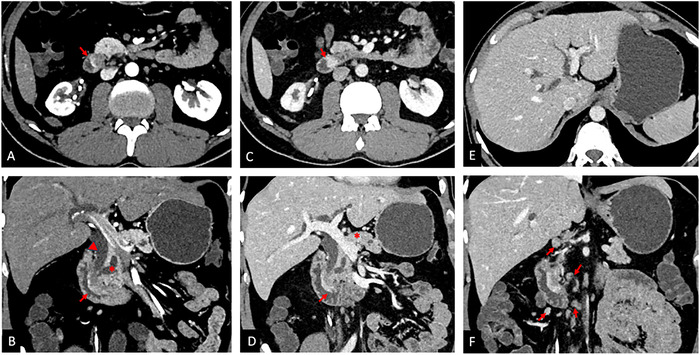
Contrast-enhanced computed tomography (A) axial reformatted image in pancreatic phase shows an ill-defined mass in the ampullary region with peripheral rim enhancement (arrow). (B) Coronal reformatted image in pancreatic phase shows a smooth bulge into the medial wall of the second part of the duodenum (arrow), as well as cutoff of the distal common bile duct (arrowhead) and pancreatic duct (asterisk) with upstream dilatation (the double duct sign). (C) Axial and (D) coronal reformatted images in portal venous phase show progressive enhancement of the mass (arrows), with the mass appearing iso-hyperenhancing to the pancreatic parenchyma (asterisk in image D). (E) Axial image in portal venous phase shows bilobar intrahepatic biliary dilatation. No focal lesions were noted in the liver parenchyma. (F) Coronal reformatted image in portal venous phase shows heterogeneously enhancing subcentimetric, periportal, peripancreatic, and mesenteric lymph nodes (arrows).
